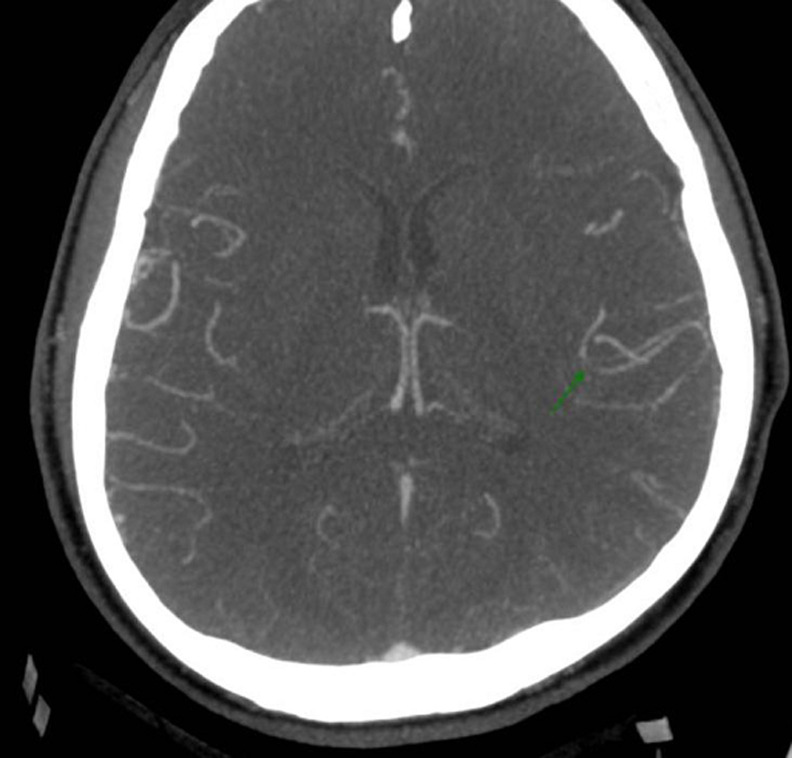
CT angiography image demonstrating partial occlusion of the left middle cerebral artery
